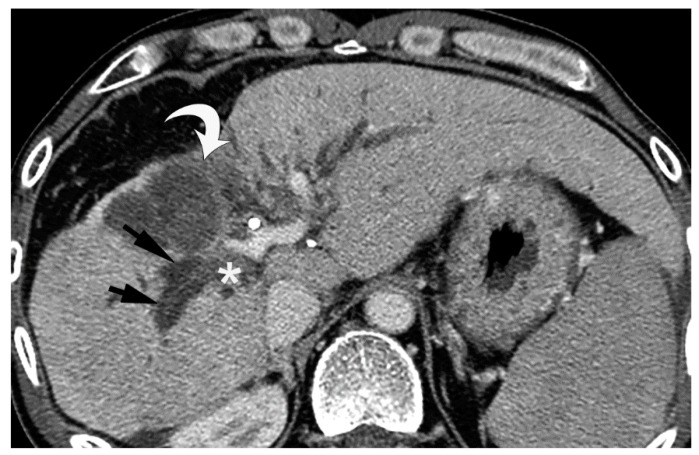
Equilibrium phase image of a contrast-enhanced CT of BDN. A hypodense tubular structure (arrows) is observed in the hepatic hilum of the right lobe. Enhancement of the right main portal branch is discontinued (*), which represents thrombotic obstruction of the portal vein caused by BDN. (Curved arrow is the biloma.).
